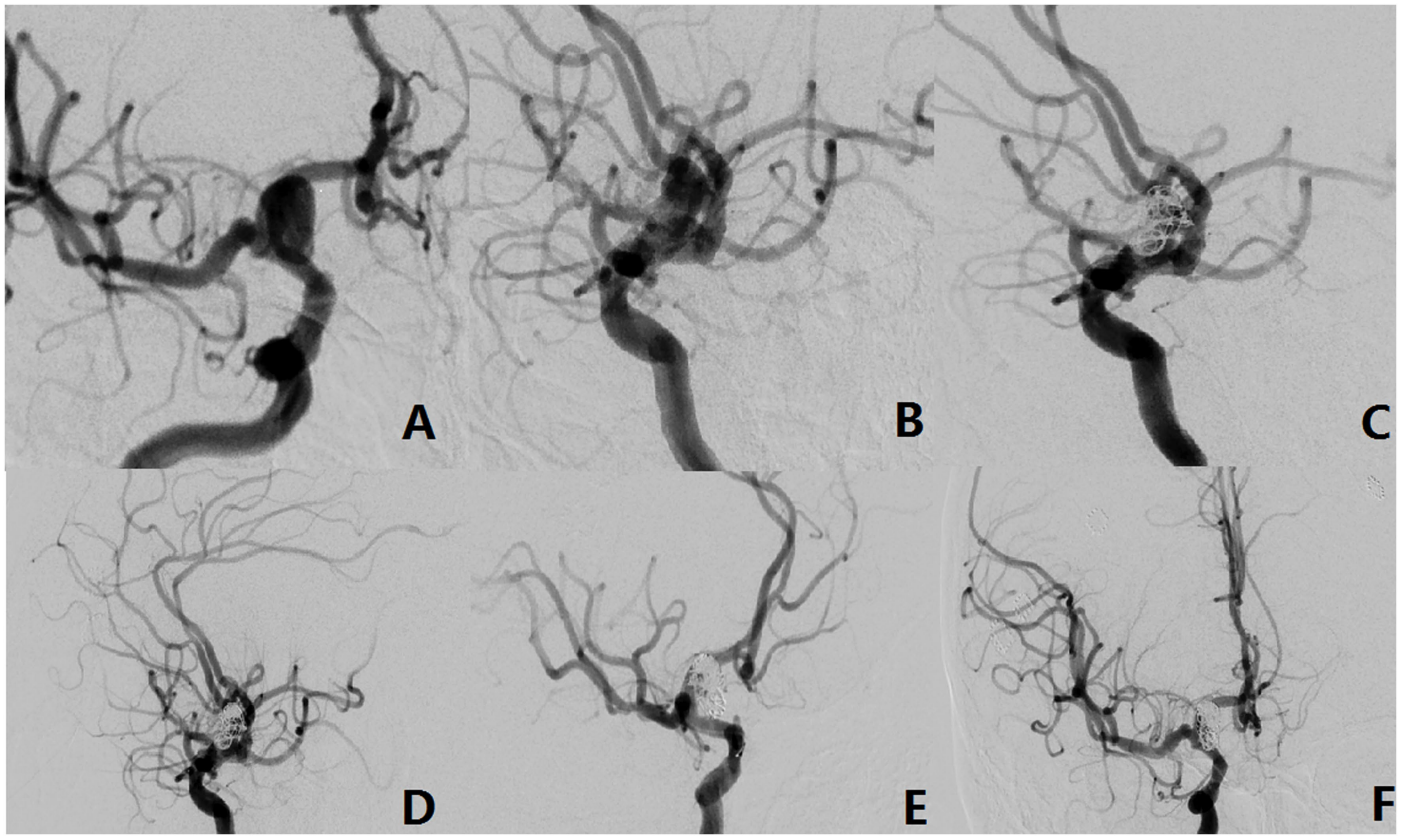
The aneurysm neck is located in the anterior wall of the parent vessel (Type-III). (A,B) A 42-year-old male with an unruptured aneurysm. The DSA showed the aneurysm originated from the anterior wall of the parent vessel. (C–E) Intraoperative situation. (F) Postoperative radiography situation.
